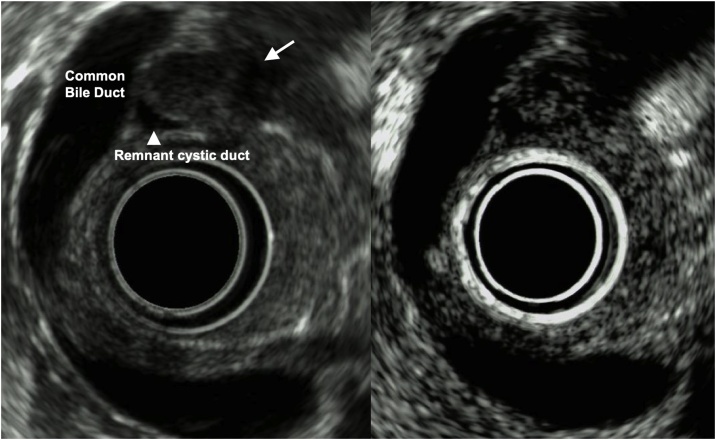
Endoscopic ultrasonography findings. Endoscopic ultrasonography demonstrated the tumor (white arrow) at the junction of the cystic duct. On Sonazoid-enhanced echo, the tumor was universally enhanced.
